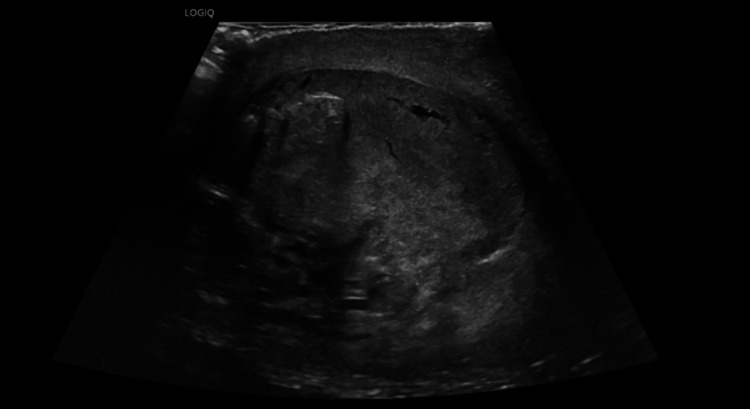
Follow-up sagittal grayscale ultrasound of the left testicle three days after injury. Demonstration of nonspecific heterogenous echotexture that is largely unchanged from the prior study. Testicular contour is normal with a continuous tunica albuginea. This examination was considered stable from the prior ultrasound examination.
